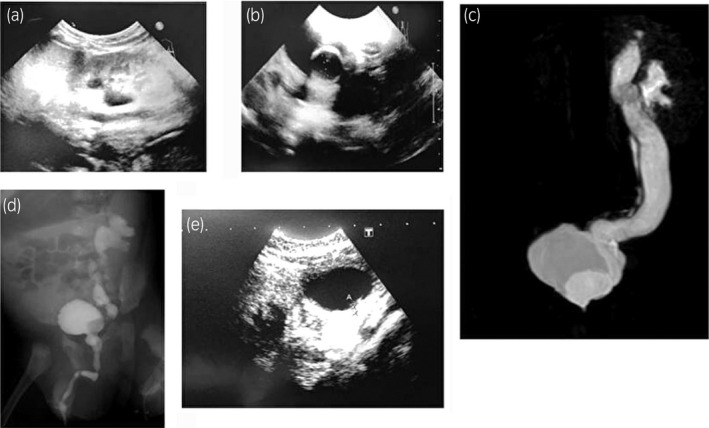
Patient 1. Ultrasonography of the left kidney (a), ultrasonography of the bladder showing a 17‐mm ureterocele (b), MRI showing ectopic ureterocele (c), VCUG showing Grade IV VUR in the left inferior kidney (d), and ultrasonography of the bladder after 38 months of conservative treatment showing reduction of the ureterocele (e).
